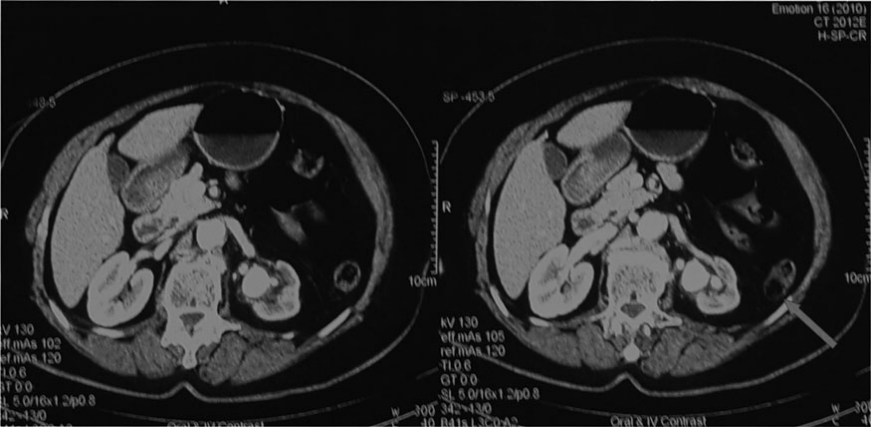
CT cross section slides showing large UPJ stone, thinning of left renal cortex, and renal pelvis dilation. *Red arrow* shows part of colon located beside left kidney (retro renal colon).
